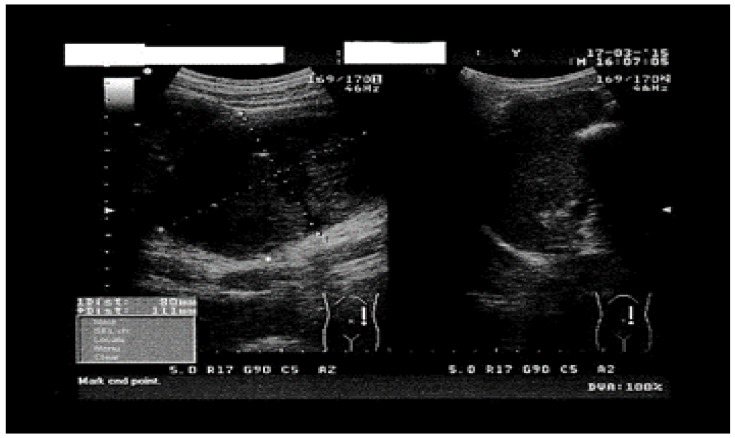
Abdominal ultrasound showing round macronodular image.
